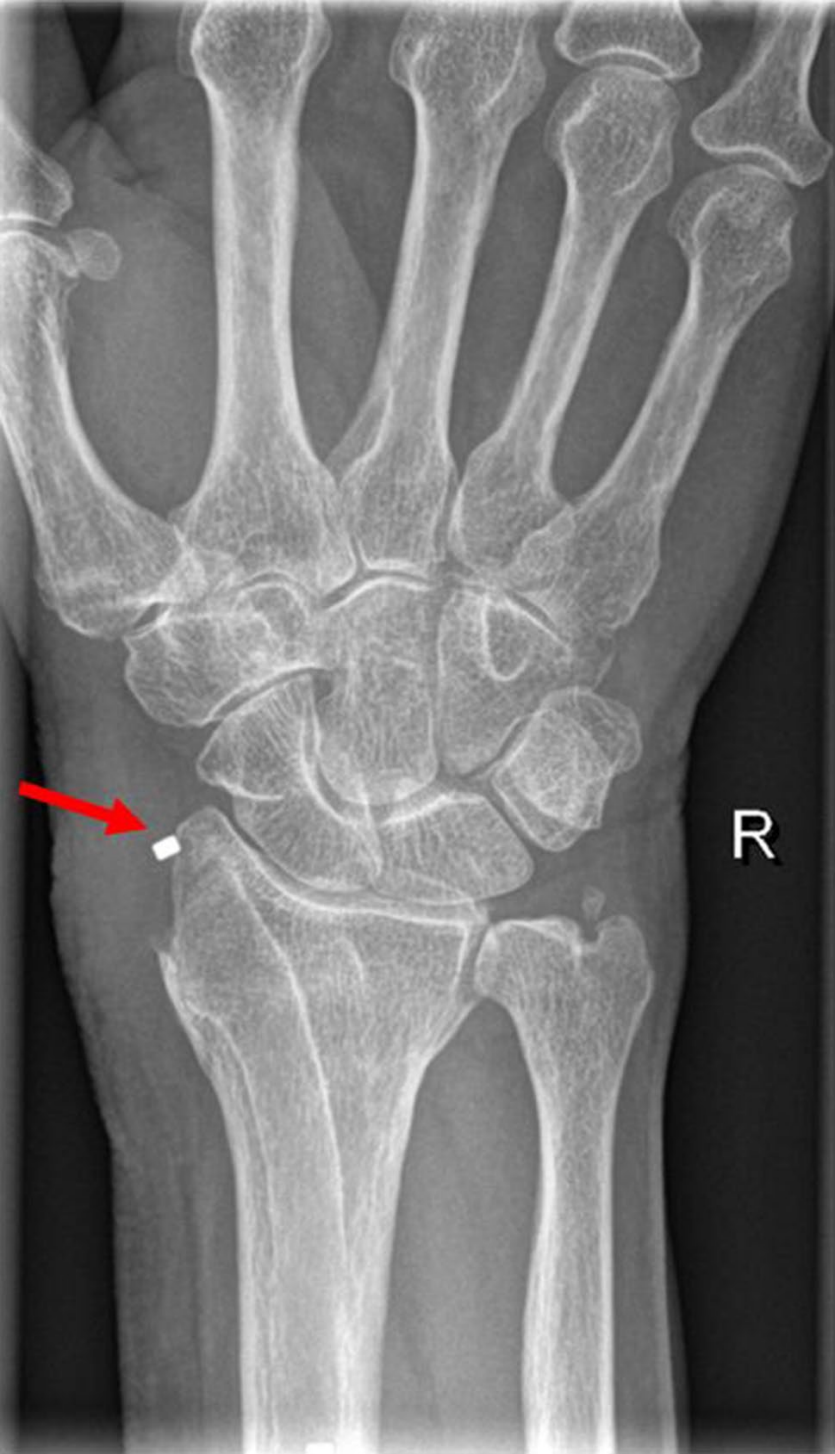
PA-radiograph at one year and 4 months after primary surgery. A small part of the implant is sticking out just proximal of the radial styloid, access point to the intramedullary canal during primary operation
